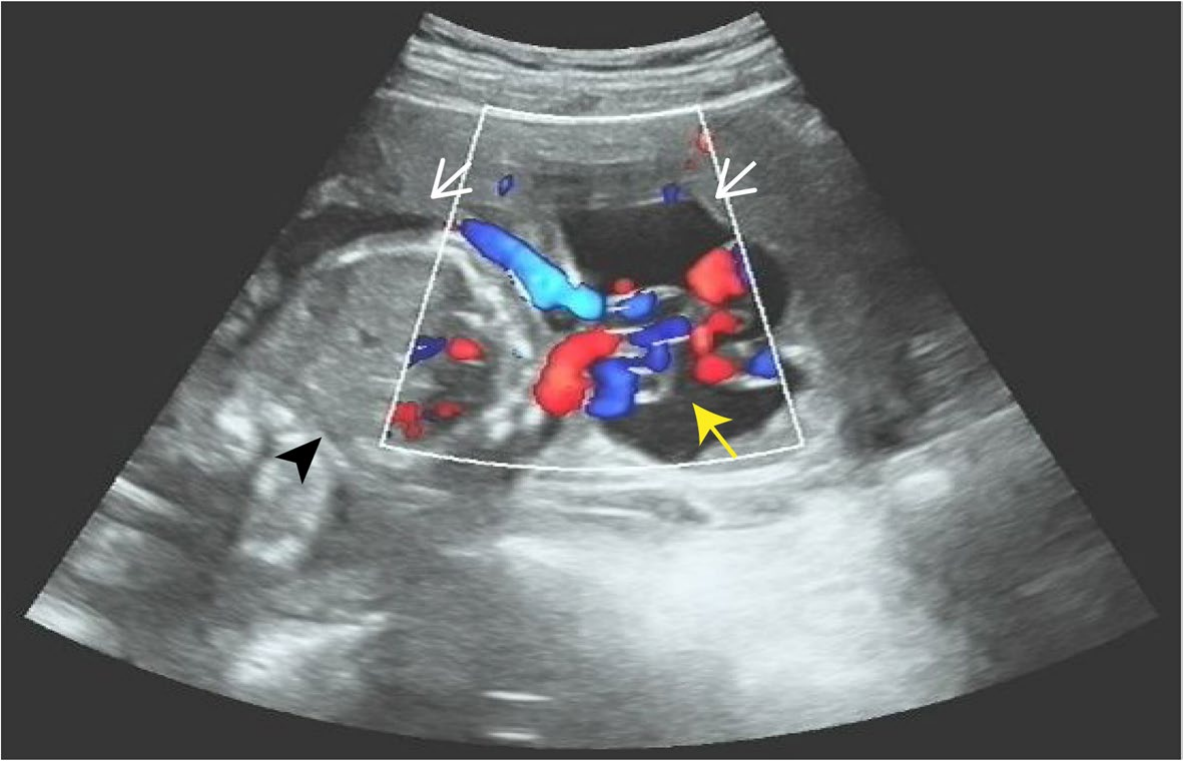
Ultrasonography images show the amniotic sac stretches across two hemicavities (white arrows). The fetus (black arrow head) is located in the right hemicavity with little amniotic fluid, whereas the umbilical cord (yellow arrow) extends to the contralateral hemicavity with most amniotic fluid
